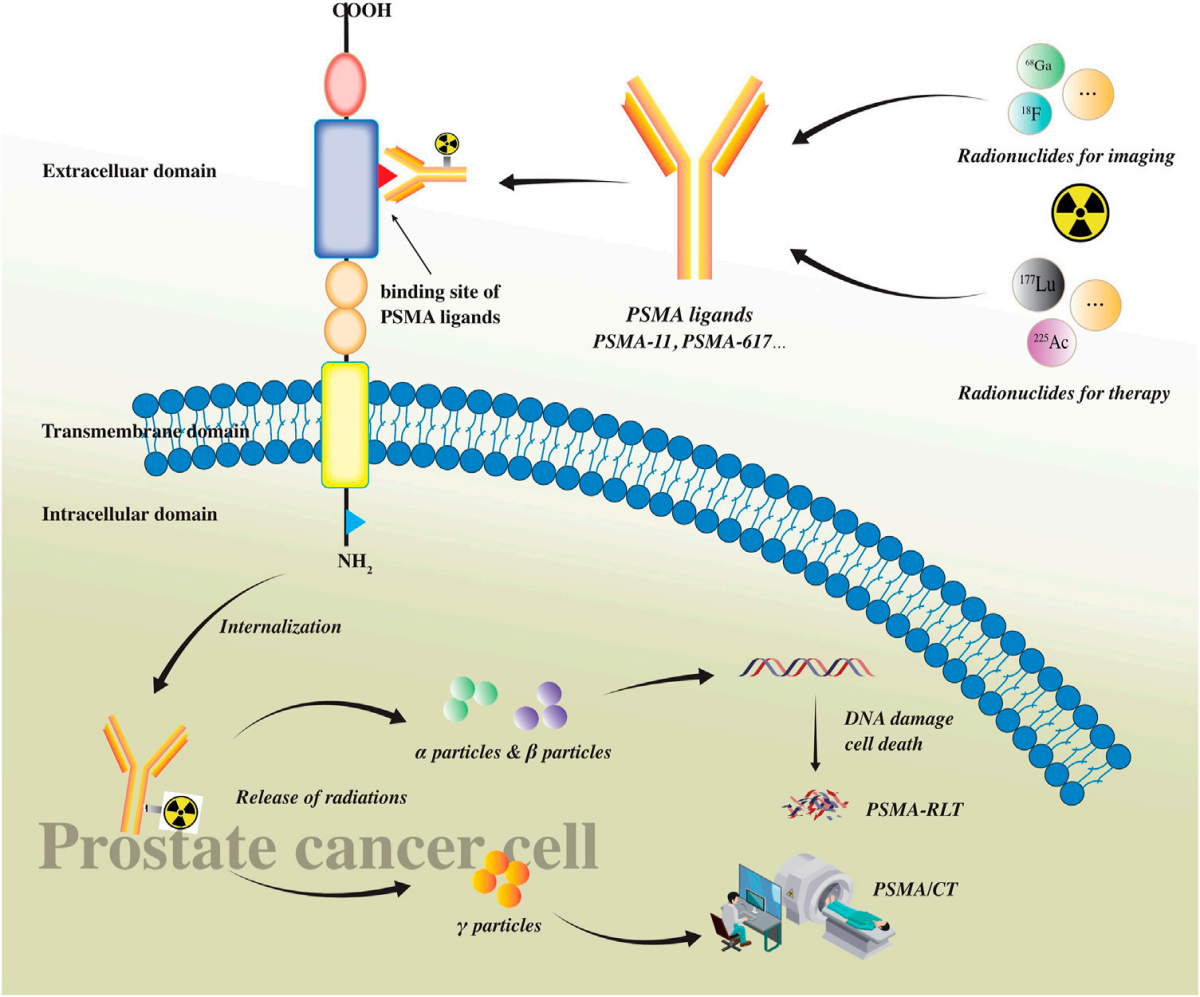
PSMA as a transmembrane glycoprotein consists of three parts: intracellular, transmembrane, and extracellular domain. There is a ligand binding site in the extracellular domain where ligands bind with PSMA on prostate cancer cells. PSMA ligands (such as PSMA-11 and PSMA-617) can be labeled with radionuclides for imaging (such as ^68^Ga and ^18^F) and/or therapy (such as ^177^Lu and ^225^Ac). After radiolabeled PSMA ligands bind with the extracellular ligand binding site of PSMA, they would be internalized into prostate cancer cells, releasing different particles. Alpha(α) and beta(β) particles can cause DNA damage leading to cancer cell death and be used in PSMA RLT. Gamma (*γ*) particles can be detected using PET scans and be used in PET/CT imaging.
